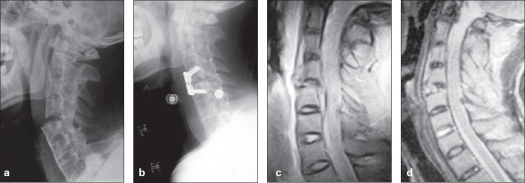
(a) X-ray of cervical spine (lateral view) of a 42 year-old male patient with ankylosing spondylitis shows C_4-5_ fracture and dislocation. (b) Postoperative X-ray of cervical spine showing anterior decompression, fusion and fixation with a plate, (c) Preoperative mid saggital T2WI of MRI shows cord compression, (d) Postoperative mid saggital T2WI of MRI shows the adequacy of reduction
